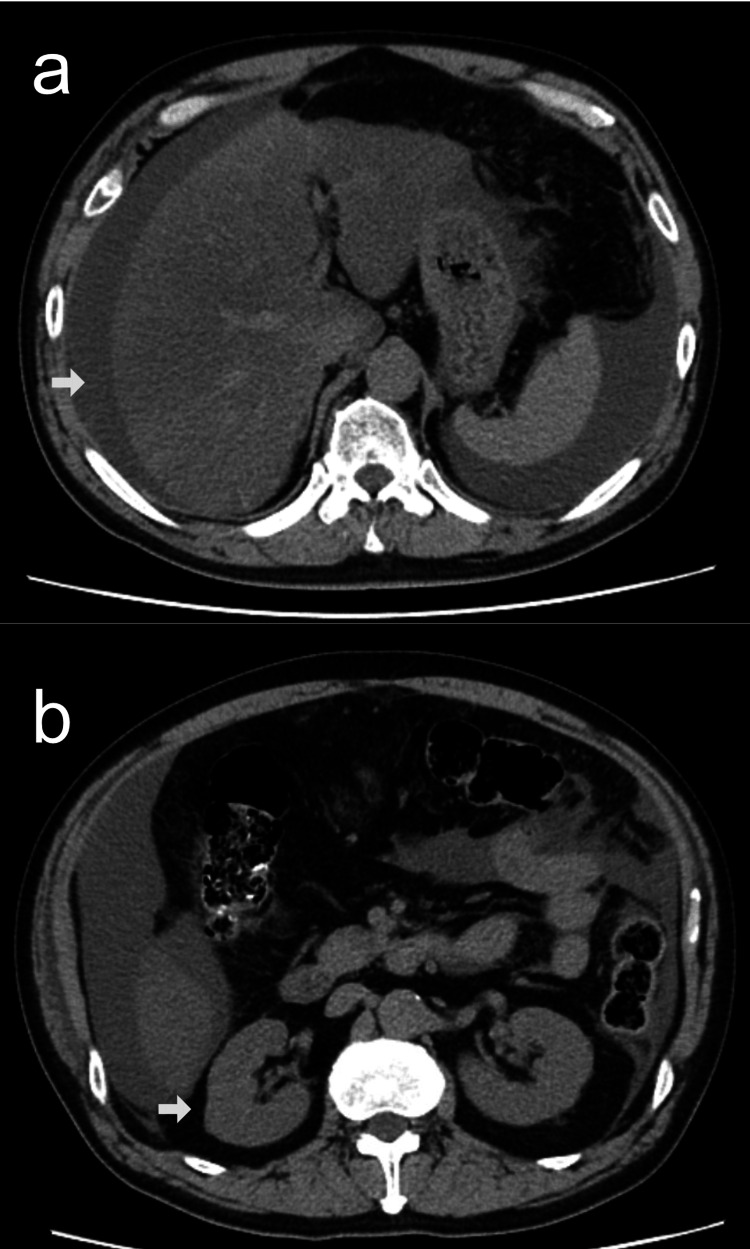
Abdominal axial CT scan on admission. Ascites and liver atrophy (a), with no apparent abnormalities in the kidneys (b).
